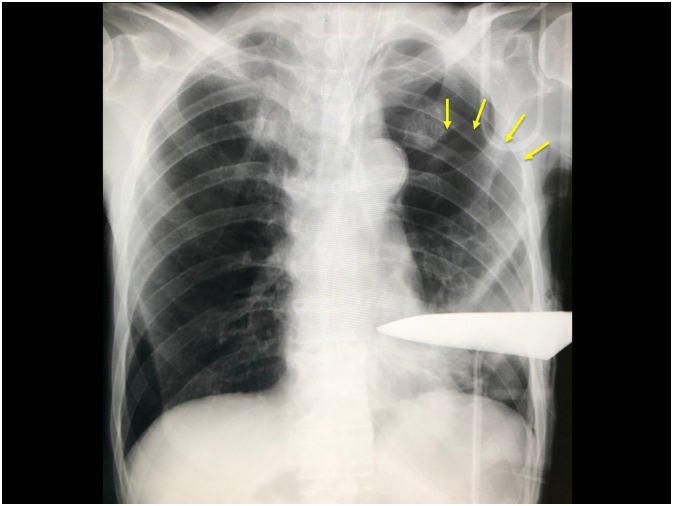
Chest radiography showing a knife penetrating the left chest wall and pneumothorax. The yellow arrows indicate the collapsed left lung. (For interpretation of the references to colour in this figure legend, the reader is referred to the web version of this article.)
